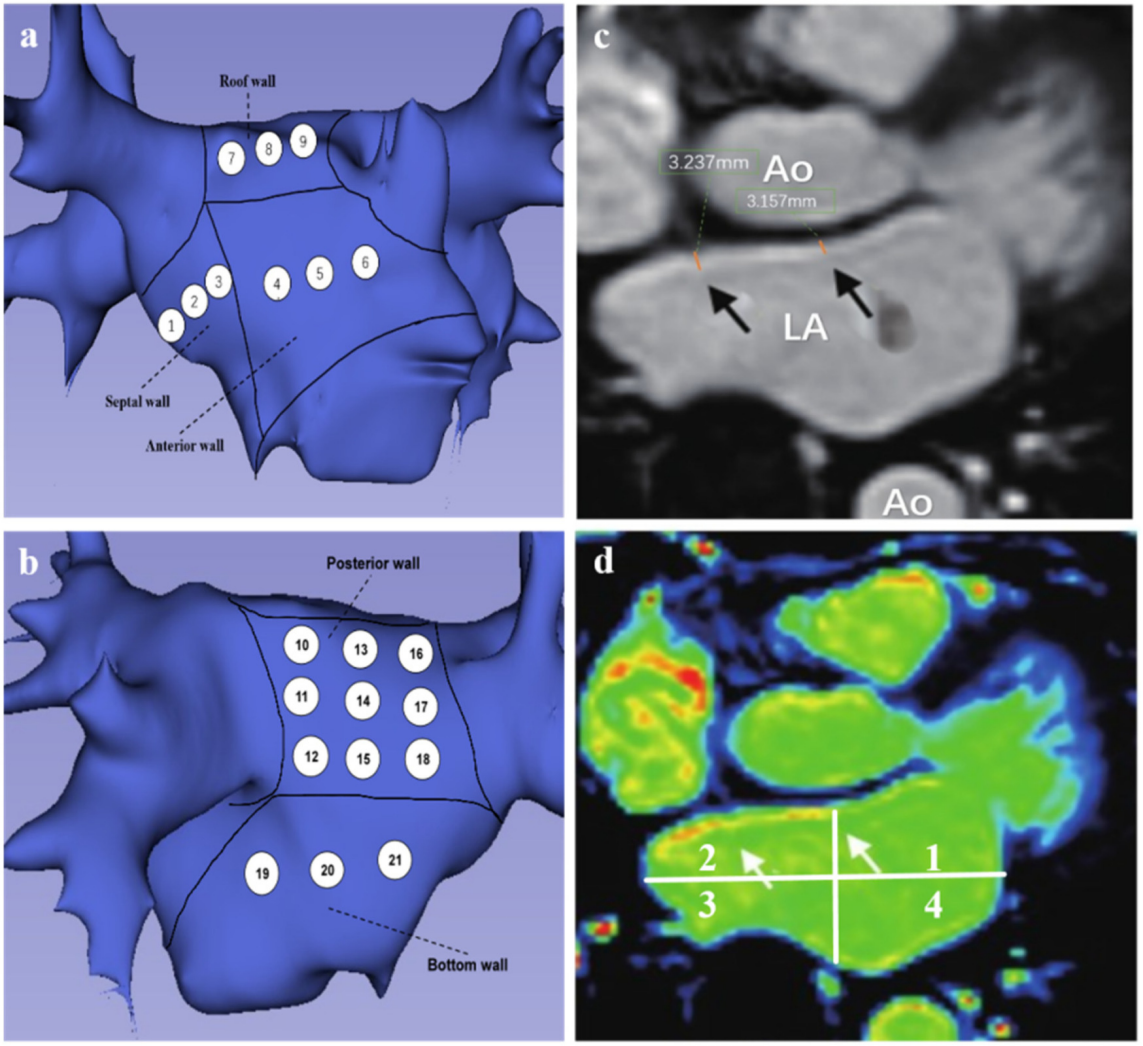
(a,b) Patterns of left atrial segment measurements. (a) Left atrial anteroposterior position, 1–3 represents the region of interstitial wall thickness measurement, 4–6 represents the region of AWT measurement, and 7–9 represents the region of RWT measurement. (b) Posterior-anterior position, 10–18 represents the PWT measurement region, 19–21 represents the BWT measurement region. (c,d) Representative LGE-MRI images of the left atrium. (c) Black arrows are examples of LAWT measurements. (d) magnetic resonance image of delayed enhancement zones (axial position), white arrows point to red and yellow zones representing fibrotic zones.
